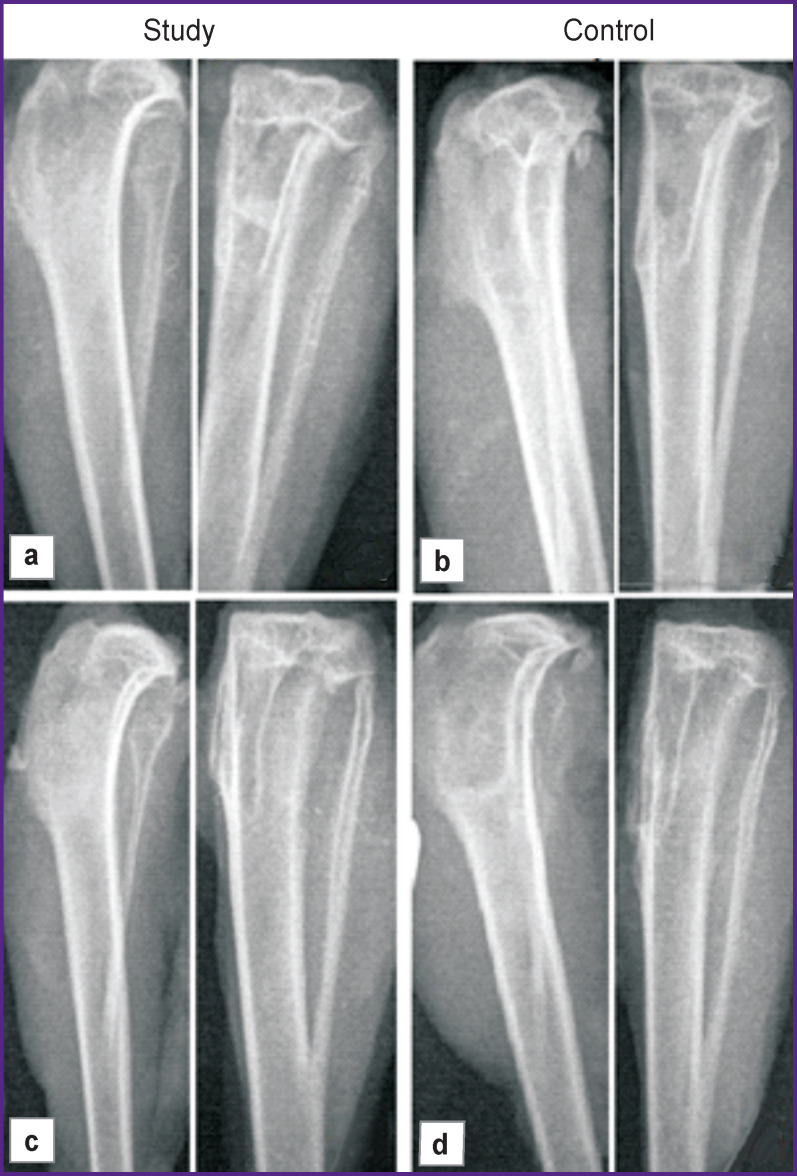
X-ray scan of the operated area: (a), (c) 30 and 60 days after implantation of autologous MSCs in the collagen matrix; (b), (d) 30 and 60 days after implantation of the collagen matrix only (control)
